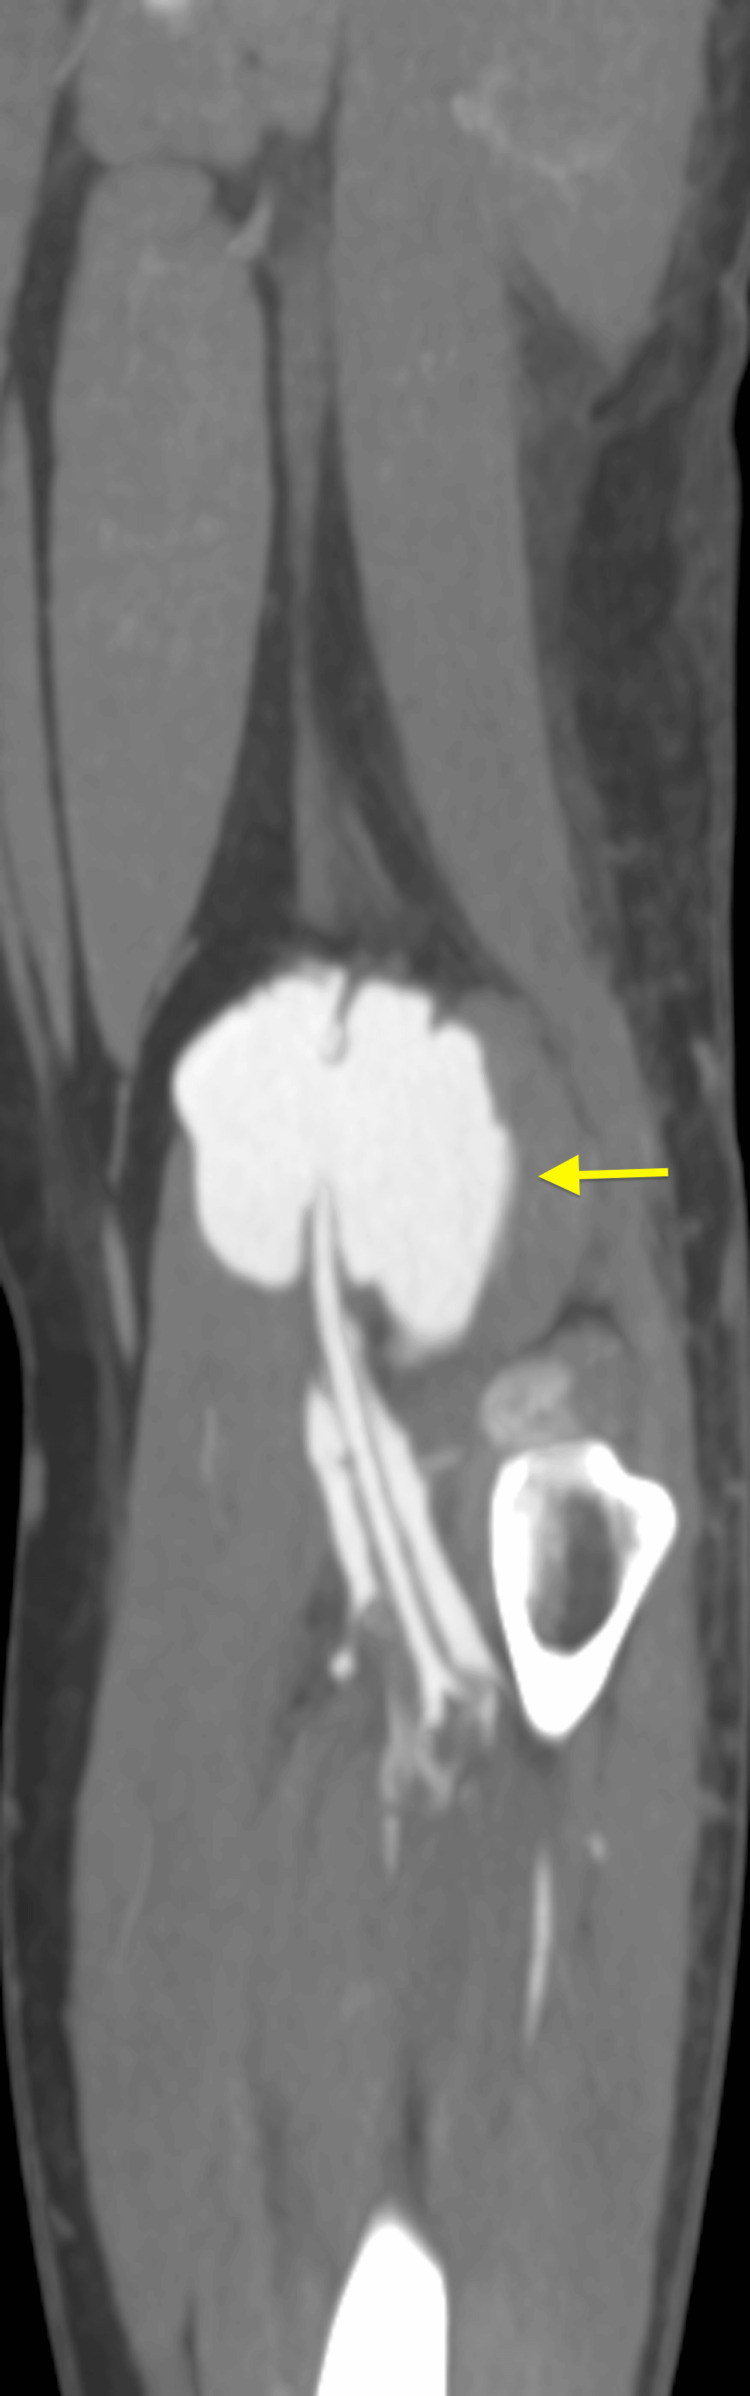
Computed tomography angiogram of the left knee with contrast (coronal view) showing popliteal vein dilation (yellow arrow).
